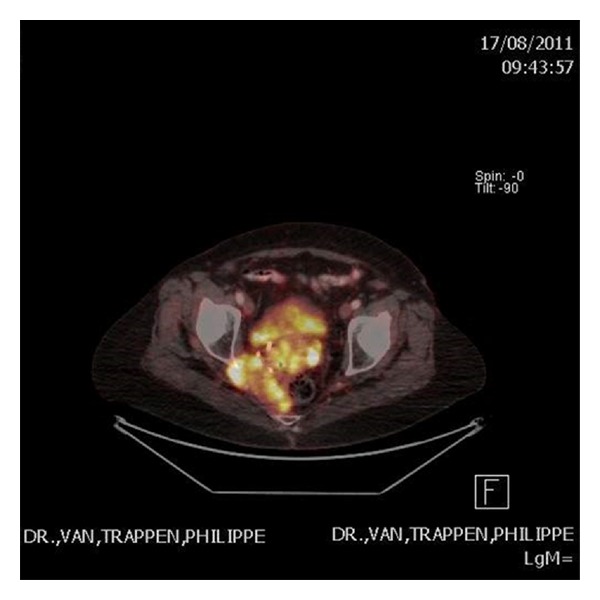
PET-CT visualizing FDG accumulation in the extranodal tumor located in the pelvis with tumor extension into the sacrum and the gluteal/obturator muscle on the right side.
